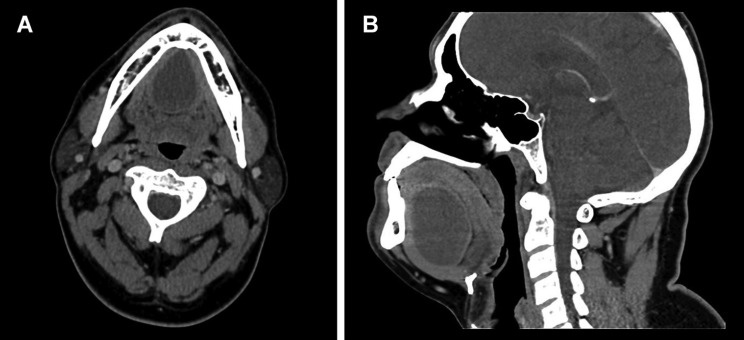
CT scan showing a well-circumscribed lesion in the sublingual space. a) Axial view. b) Sagittal view.
